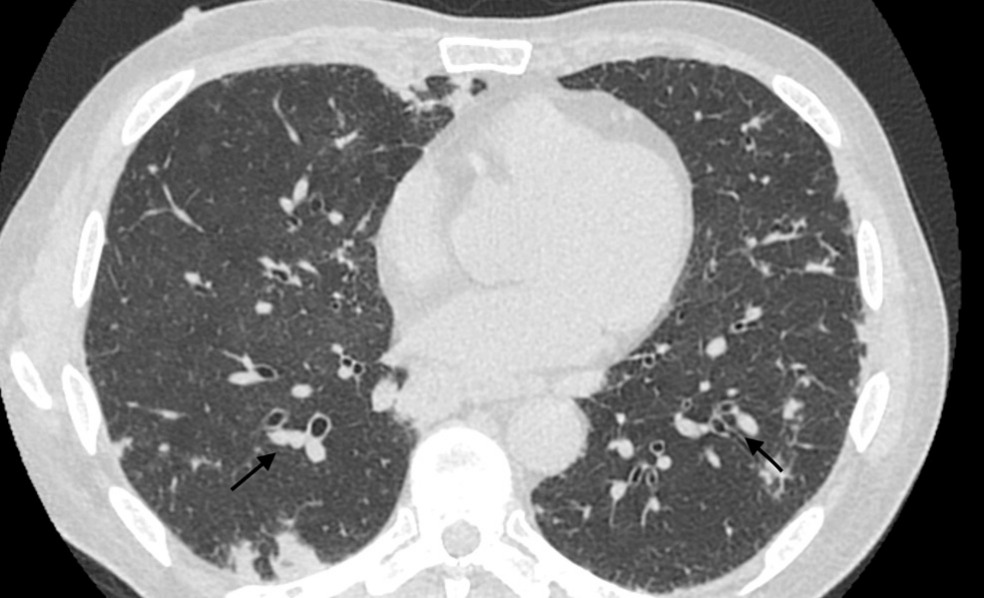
Initial computed tomography without contrast demonstrating bilateral nodular densities (black arrows), as well as mediastinal and hilar lymphadenopathy.
